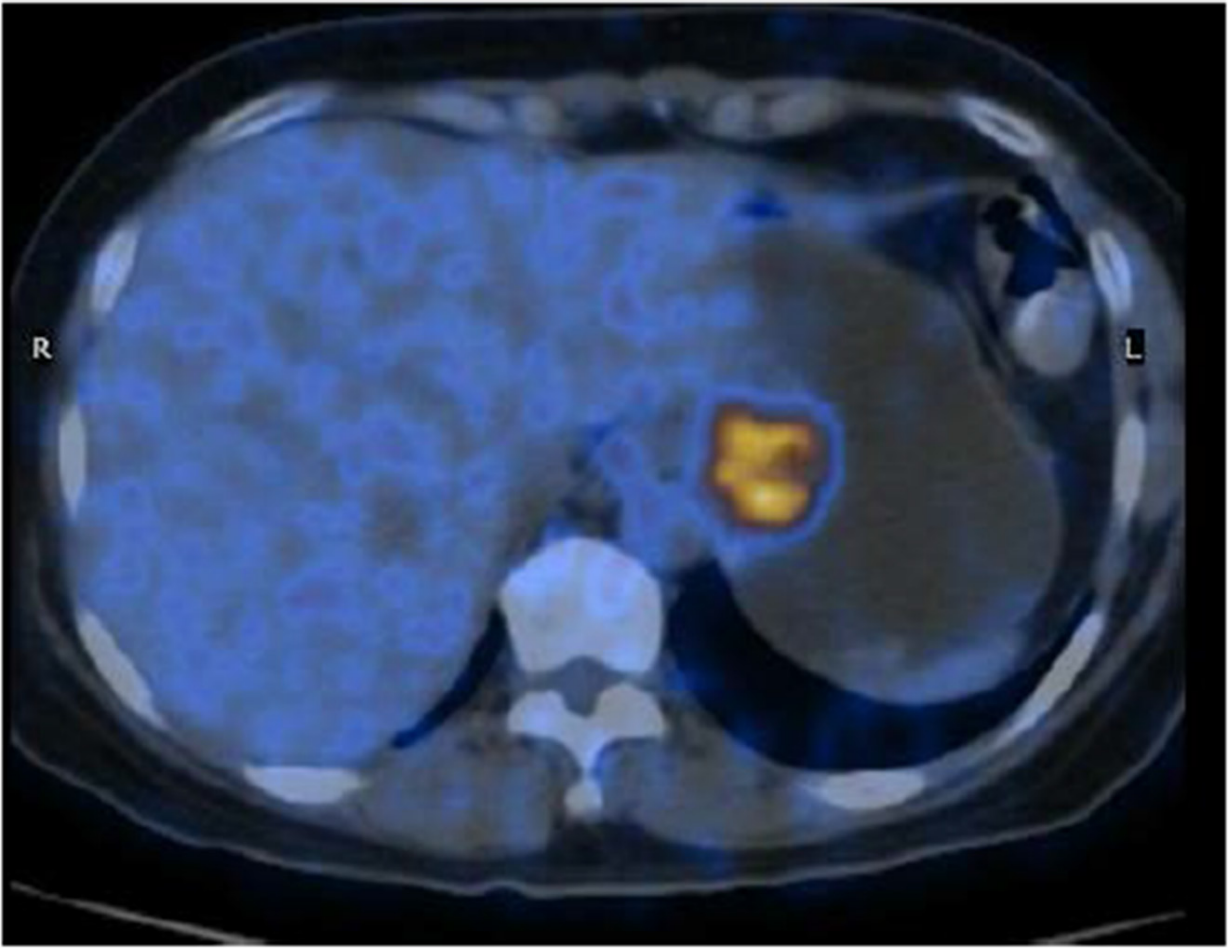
PET/CT scan showing the gastric lesion in the cardia
